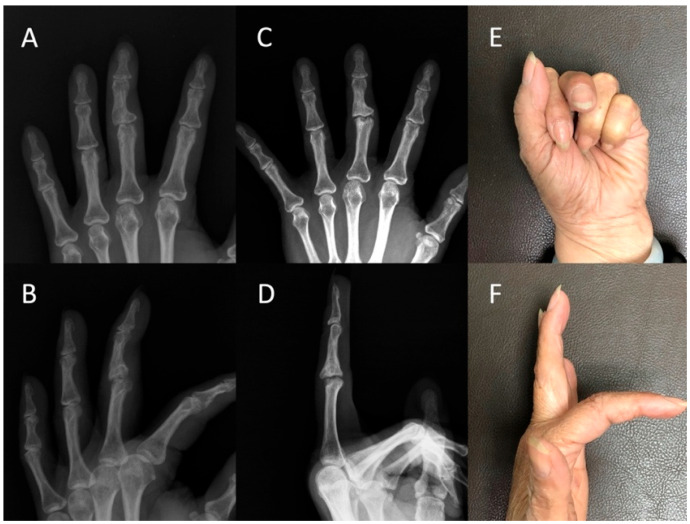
Case #8: A 57-year-old woman developed left middle finger post-traumatic osteoarthritis. Radiographs of the left middle finger in the (A) anteroposterior (AP) view and (B) lateral view revealed post-traumatic osteoarthritis of the proximal interphalangeal joint in the preoperative status. The five-year follow-up assessment demonstrated the preservation of joint space in the (C) AP and (D) lateral views. The active flexion of the finger was 40° (E) with no extensor lag (F).
